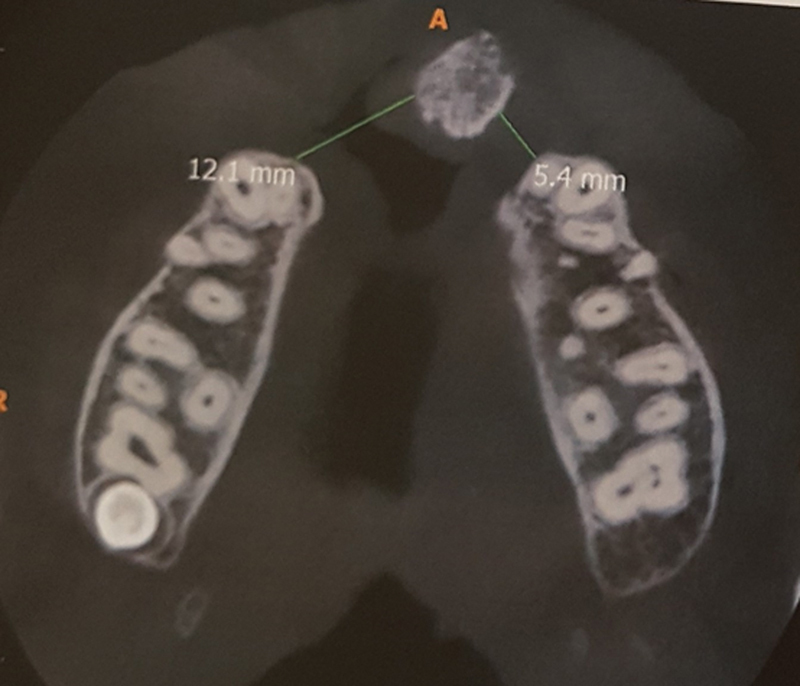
Bone volume estimation measurements of alveolus cleft by CBCT, 6-month postoperatively in the follow-up period. CBCT, cone beam computerized tomography.
